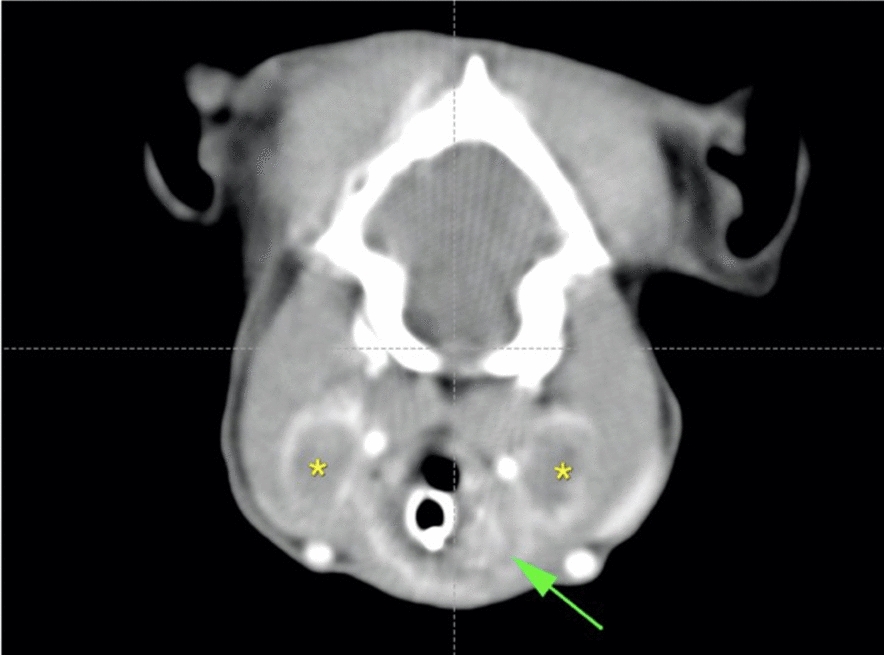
Contrast enhanced CT scan of the head: post contrast CT image of the lesion showing the moderately contrast enhancing laryngeal mass (arrow), and bilaterally enlarged retropharyngeal lymph nodes (asterisks)
